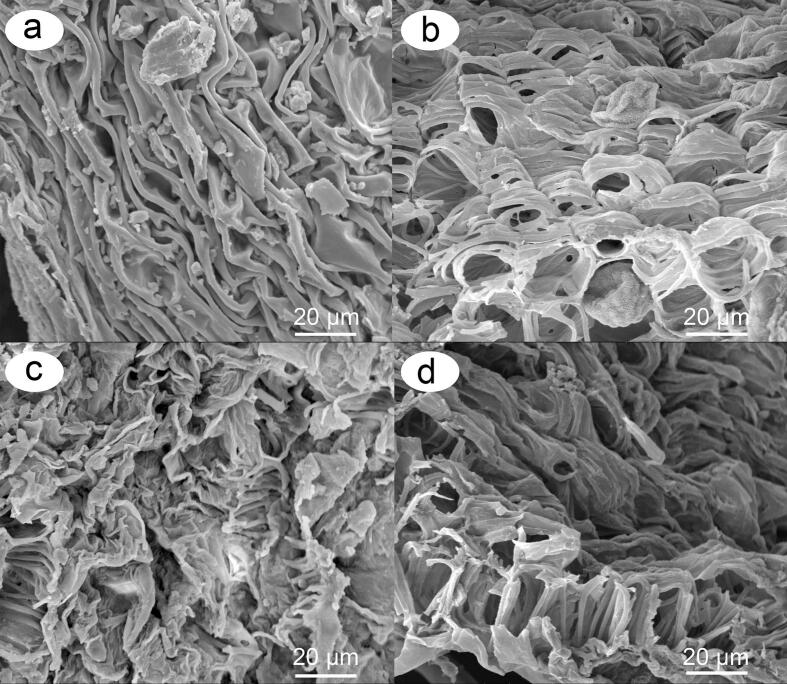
SEM images of male inflorescences of *Populus alba* × *berolinensis* before and after treatment. Raw sample (a); ultrasonic treatment (ultrasonic irradiation power: 200 W, ultrasound irradiation time: 30 min) (b); cellulase hydrolysis treatment (incubation temperature: 50 °C, incubation time: 120 min) (c); and ultrasound-assisted cellulase hydrolysis treatment (ultrasound irradiation power: 200 W; ultrasound irradiation time: 30 min; incubation temperature: 50 °C; incubation time: 120 min) (d).
